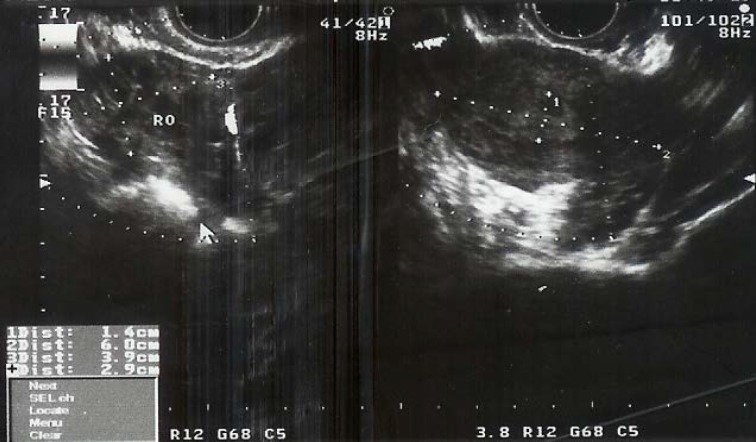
The ultrasound of patient that shows suspicious mass in right adnex (white arrow).
